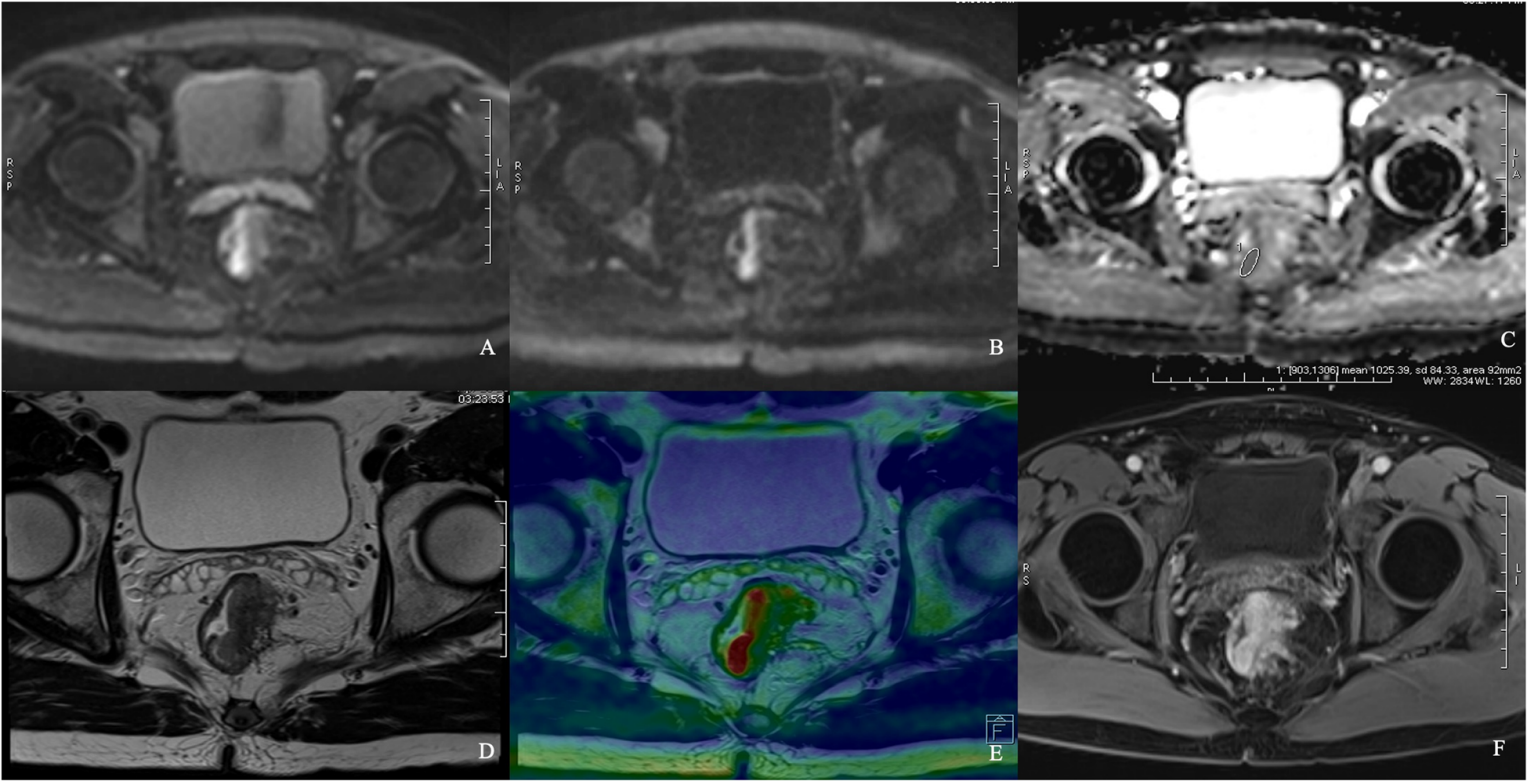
iShim DWI for patient with primary rectal cancer of Grade 1. DW images of b = 800 s/mm^2^ (a), b = 1600 s/mm^2^ (b), ADC map (c), T2WI (d), infusion images of both T2WI and DWI (e) and dynamic T1WI (f) showed the same location of lesion. Average ADC value measured was 1025.39 × 10^− 6^ mm^2^/s (c)
